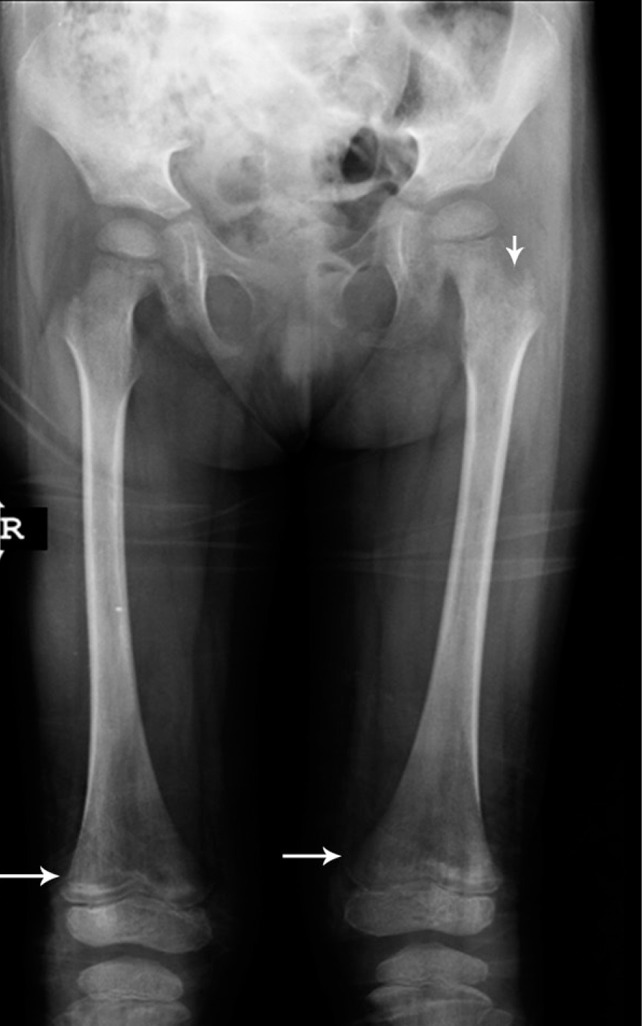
A 6-year-old girl with AML presenting with weakness, pallor and tenderness of lower extremities. Radiography reveals metaphyseal hypodensity on both femurs with sclerotic lesions (arrows). There are also lytic lesions in the left femoral neck (arrowhead).
